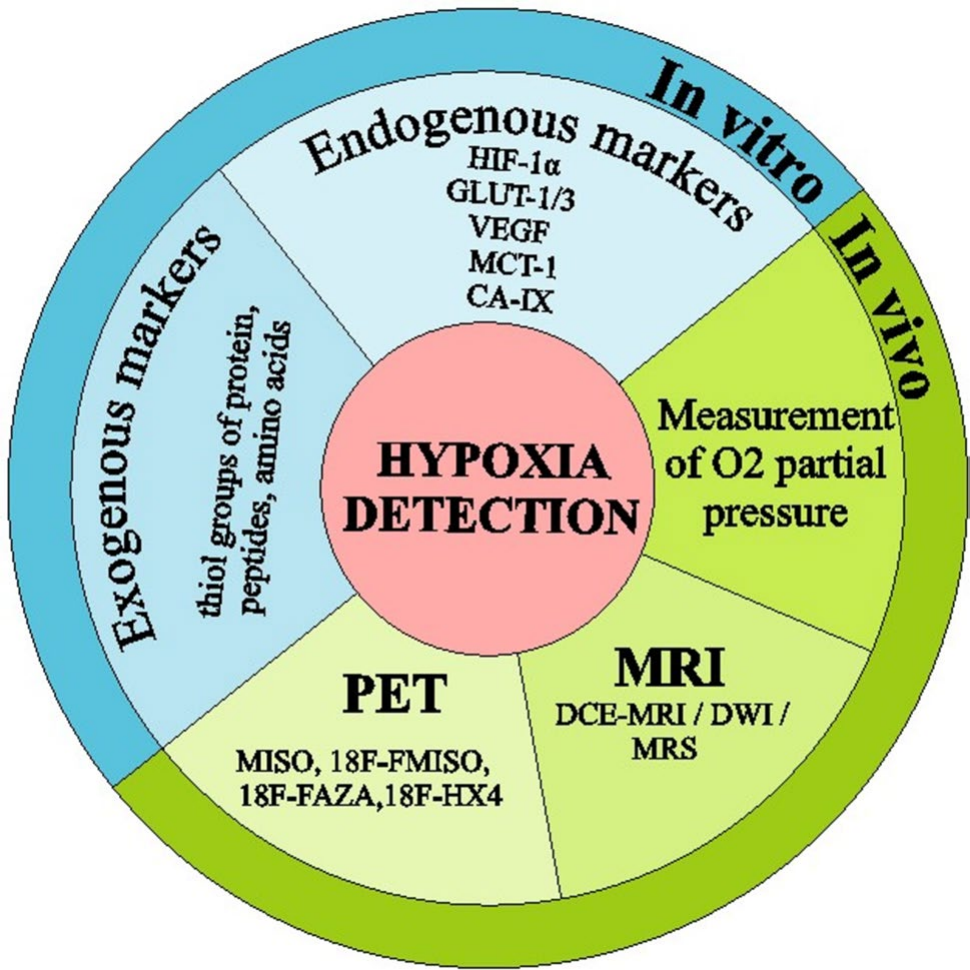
Detection of hypoxia in tumors. Hypoxia in tumors can be detected in vitro by determination of exo- and endogenous markers and in vivo by measurement of O_2_ pressure, and MRI and PET imaging
